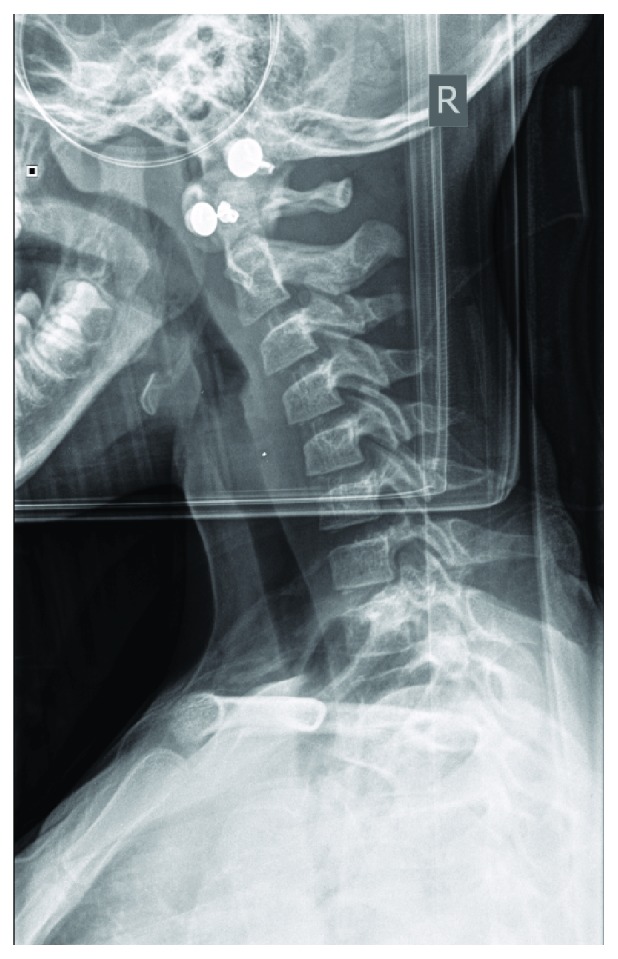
Lateral C-spine radiograph.
